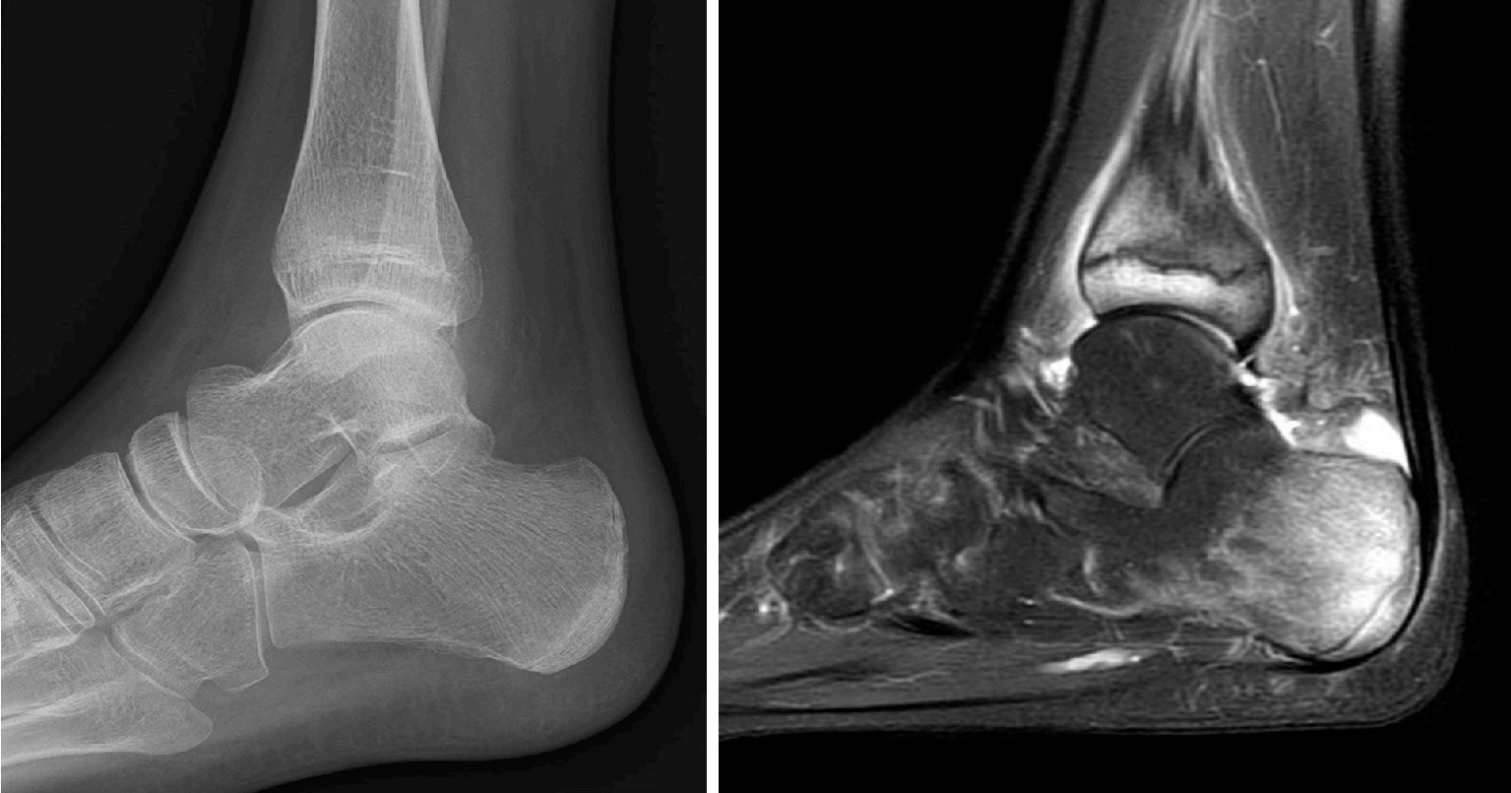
Radiograph and MRI of the heel performed 1 year and 6 months after the initial visit. MRI revealed bone marrow edema, despite no abnormalities being observed on plain radiography. MRI: magnetic resonance imaging
